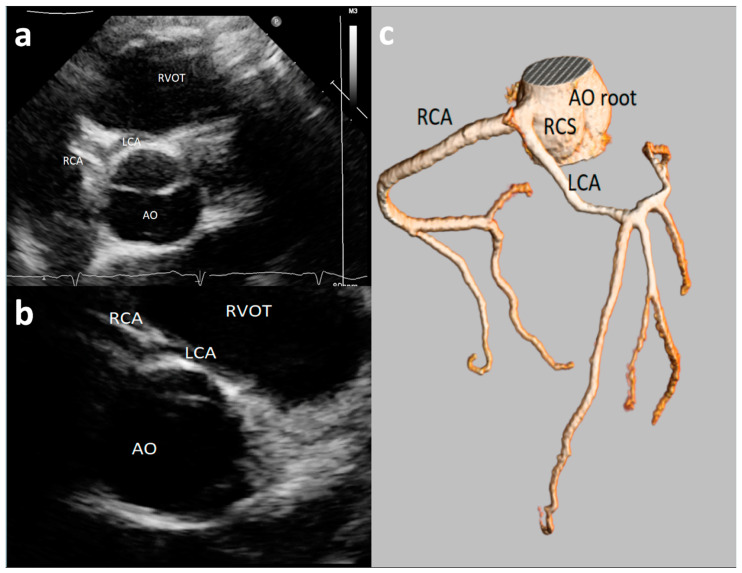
An asymptomatic 14-year-old boy evaluated with negative stress test for murmur at routine soccer screening. Origin of the left common artery (LCA) from the right sinus of Valsalva was incidentally discovered at echocardiography ((a,b)—short-axis view with slightly different angulation) and confirmed by Coronary Computed Tomography Angiography (CCTA) (c). RCA = right coronary artery; LCA = left common artery; RCS = right coronary sinus; AO = aortic root; RVOT = right ventricle outflow tract.
